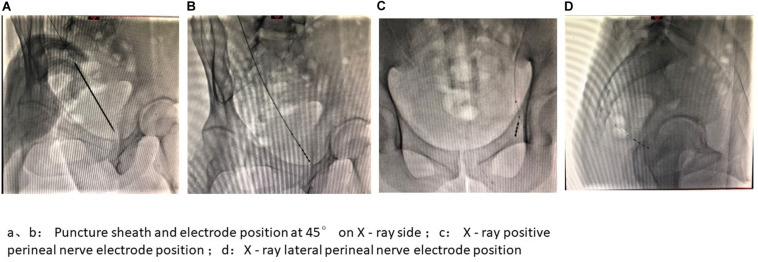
Intraoperative image presentation of PNM I. (A,B) Puncture sheath and electrode position at 45° on X-ray side; (C) X-ray positive perineal nerve electrode position; (D) X-ray lateral perineal nerve electrode position.
